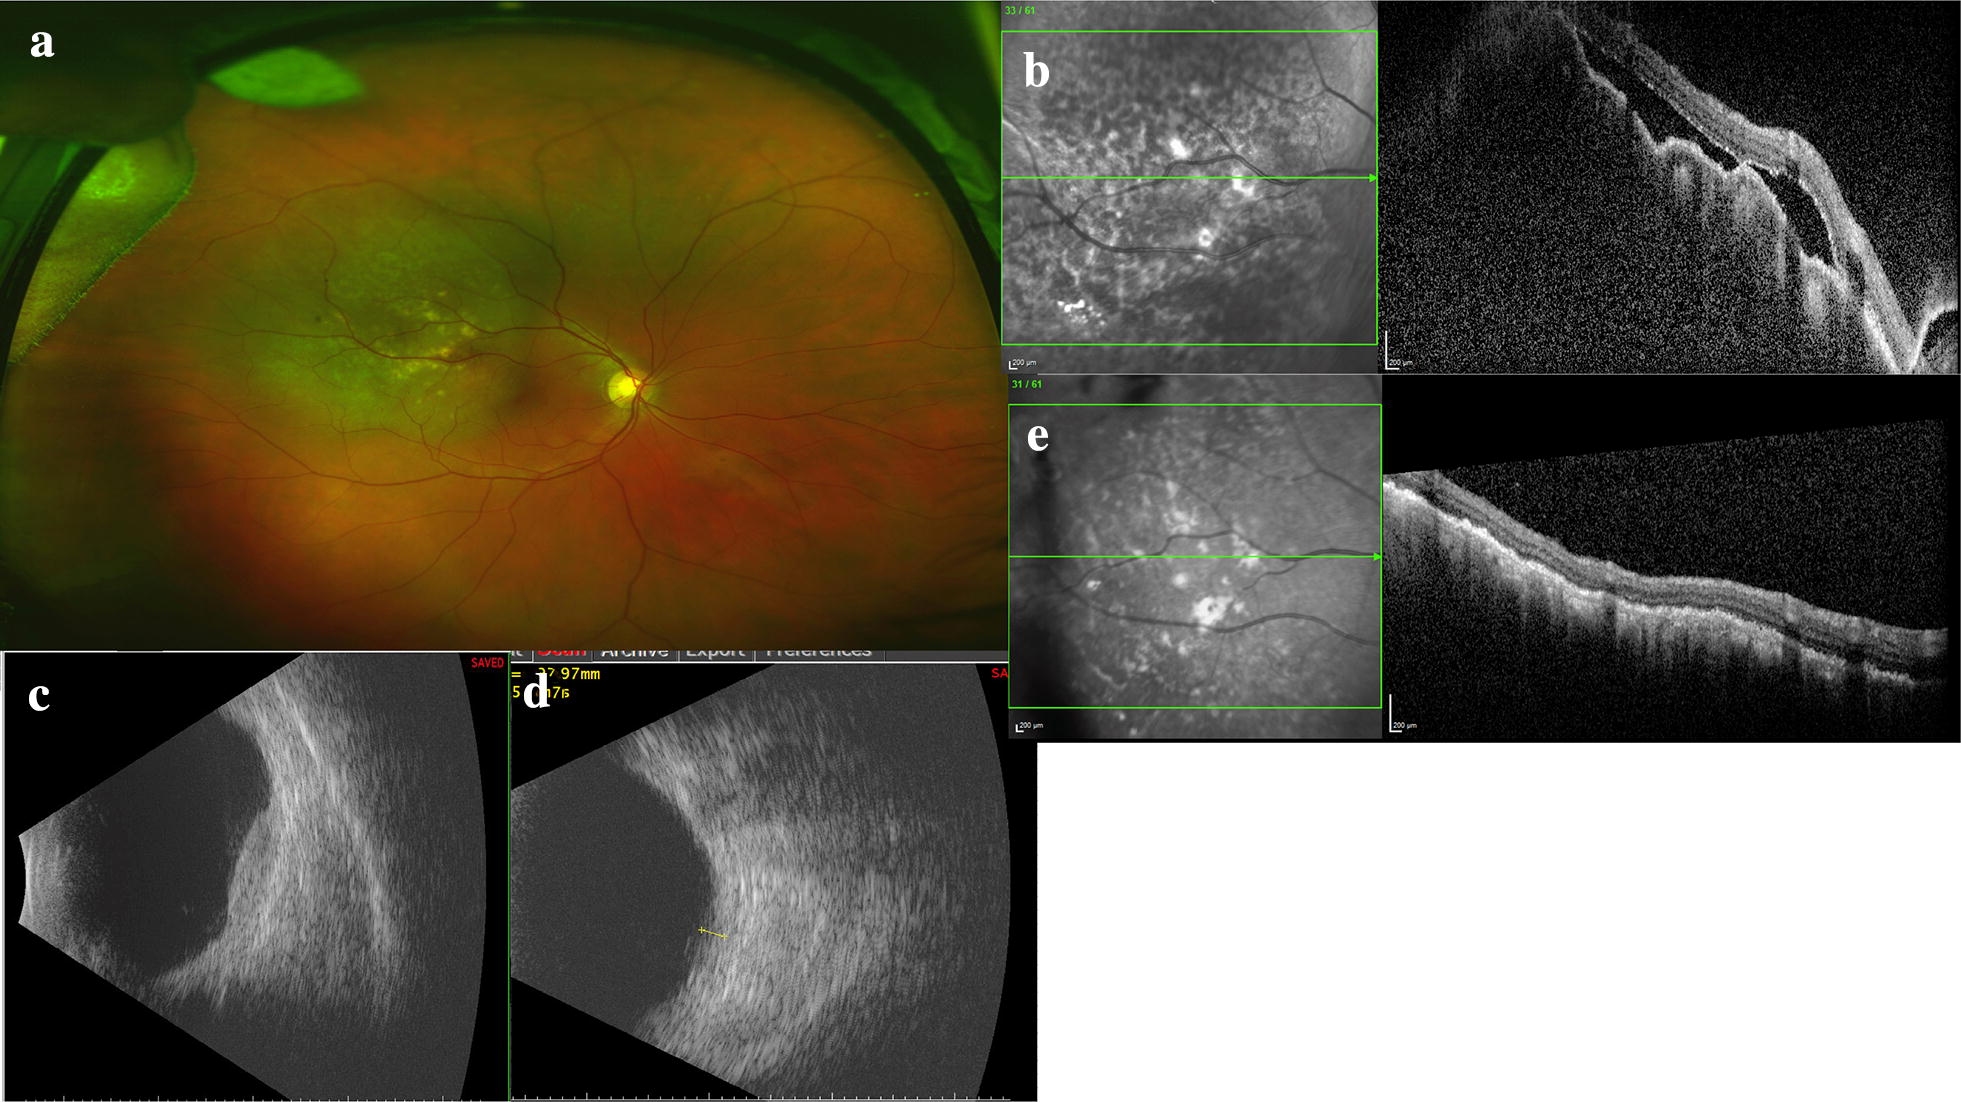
Multimodal imaging of a uveal metastasis from lung adenocarcinoma. The patient presented with reduced vision in his right eye and clinical examination revealed a large, placoid-like mass at the superotemporal arcade with overlying pigmentary changes and associated fluid. a Optos wide-field imaging of the placoid lesion with overlying pigmentary changes. b OCT with en face image with pigmentary changes, irregular, low-lying choroidal mass and resulting undulations of the choroid and associated subretinal fluid. c Ultrasonography reveals the lesion is irregular. d After target treatment the lesion thickness decreases on ultrasonography and e the choroidal mass has resolved and the subretinal fluid absorbed
